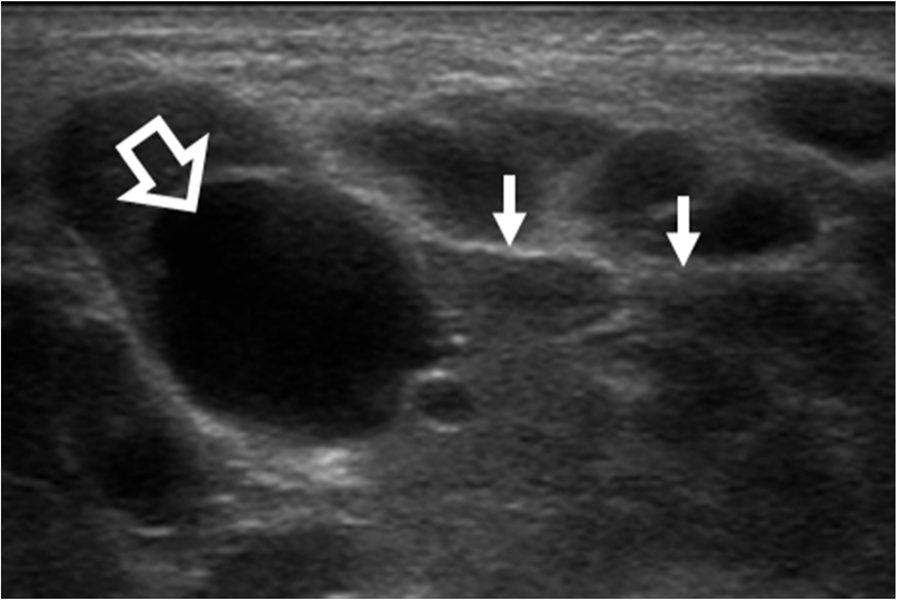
Ultrasound of a 33-year-old woman with parotid MALToma presenting as bilateral parotid gland swelling for 7 years. The parotid gland shows a dominant homogeneous very hypoechoic solid nodule that could be mistaken for a cyst (open arrow) against a background of numerous small homogeneous solid hypoechoic nodules interspersed with echogenic strands (arrows)
